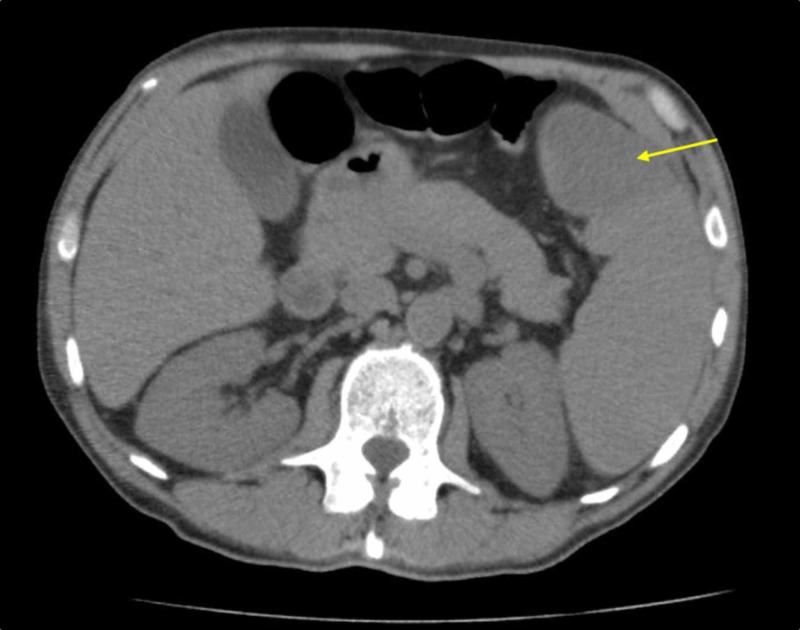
Computed tomography (CT) scan of abdomen/pelvis showing splenomegaly with a hypodense region (yellow arrow) suggestive of a splenic infarct
